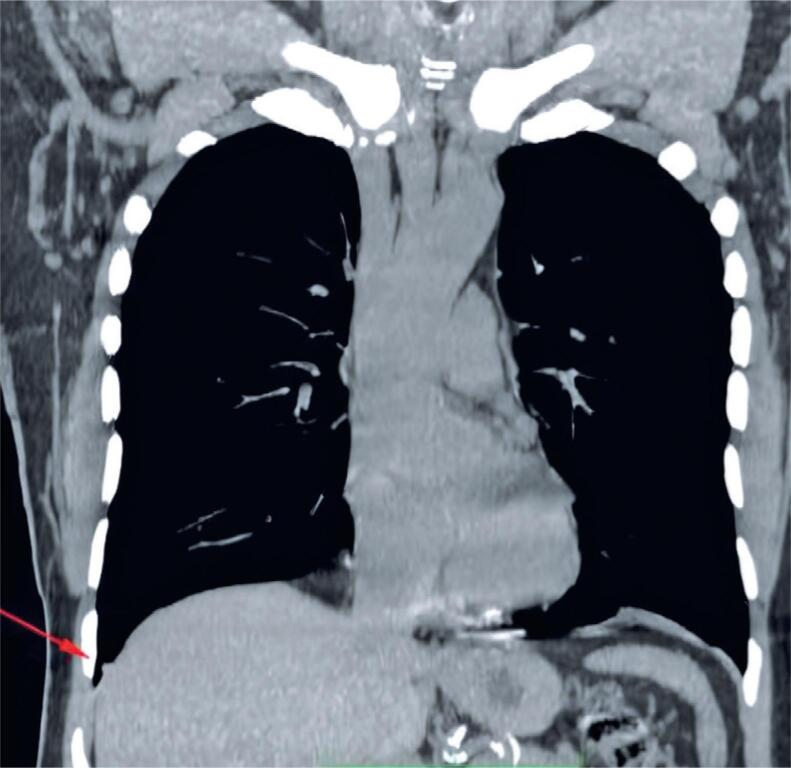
Chest CT in coronal plane. The arrow indicates mild thickening of the costodiaphragmatic pleura
